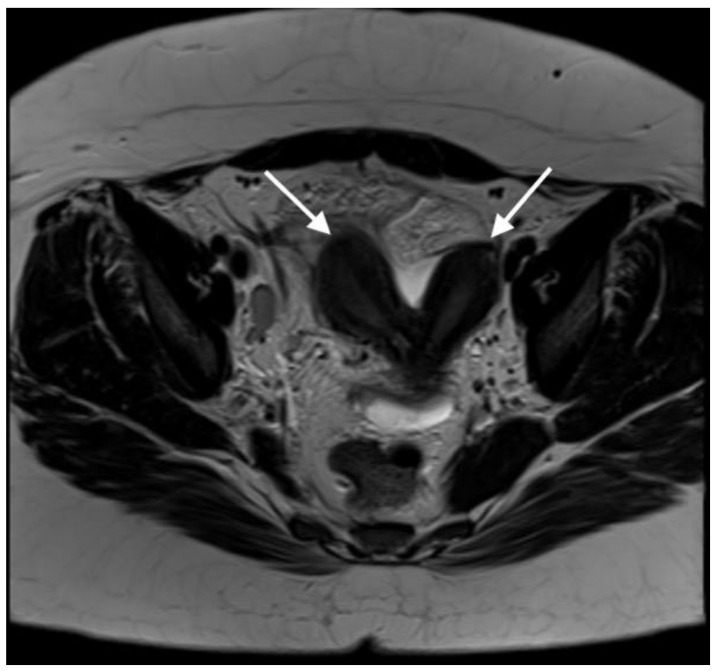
Axial T2-weighted MR image demonstrates uterus didelphys (two uterine cavities indicated by white arrows). A 21-year-old woman presented with lower abdominal and back pain, mainly located on the right side. The pain was constant and progressively worsened over a six-day period. She also reported episodes of vomiting over the last two days, constipation and a loss of appetite. Additionally, she had a previous assessment in A + E five days prior to this admission, where she was diagnosed with a chest infection and was treated with Co-amoxiclav. Her previous medical history and family history were unremarkable, she had never had any previous surgeries, had never conceived and was not allergic to any medication. Initially, she was assessed by members of the A + E team and was referred to the surgical team to exclude appendicitis or mesenteric adenitis. Upon examination, her abdomen was soft with voluntary guarding on the right iliac fossa. She was pyrexial (T: 38.1 °C), tachycardic (HR: 129 bpm), tachypnoeic (RR: 19), with normal blood pressure (BP: 119/80 mmHg) and oxygen saturation levels (SO_2_: 98%). Biochemical and haematological investigations revealed elevated CRP (>320 mg/L), white cell count (WCC: 16 × 10^9^/L) and platelets levels (405 × 10^9^/L). The liver and kidney functions were normal. Venous blood gas indicated a pH of 7.35 and lactate levels of 1.3 mmol/L. The urine sample indicated the presence of leucocytes, protein and blood. The urine pregnancy test was negative. The patient underwent a CT of her abdomen/pelvis after the initial surgical review. It revealed a large complex pelvic collection, measuring 7 × 6 × 10 cm, arising from the right adnexa, with the inflammatory process extending into the uterus. The CT also suggested incidental right renal agenesis, which the patient was not aware of. The findings were suggestive of a tubo-ovarian abscess (TOA), and the patient was started on intravenous ceftriaxone and metronidazole, as per the unit’s protocol. Due to the CT scan findings, the patient was then referred to the gynaecology team. Her gynaecological history indicated a menarche at 10 years of age. Her periods were regular, but she reported significant dysmenorrhea and heavy menstrual bleeding. Clinical examination including a speculum exam revealed that the cervix was difficult to visualise, since it was deviated to the left. A purulent foul-smelling discharge was also noted as well as a fullness on the anterior fornix, but no signs of significant tenderness were present at the time of the examination. After the gynaecological assessment, a pelvic ultrasound was requested to further characterize the pelvic mass. TVUSS was performed approximately 24 h after the CT, while the patient remained febrile. The sonographic assessment revealed the collection, previously attributed to the TOA, but the additional finding of uterus didelphys with two cervices was also noted. The combination of renal agenesis and uterine abnormality raised the possibility of a Müllerian abnormality; thus, an abdomen/pelvis MRI with contrast was requested in order to confirm the diagnosis. The MRI report confirmed that there was a double uterus and cervix and further added that there was a longitudinal vaginal septum (U3bC2V2 based on the ESHRE/ESGE classification [1]).
